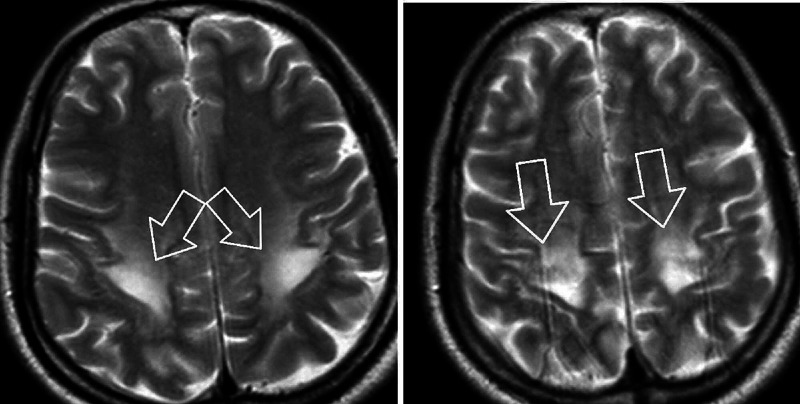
MRI brain without contrast
